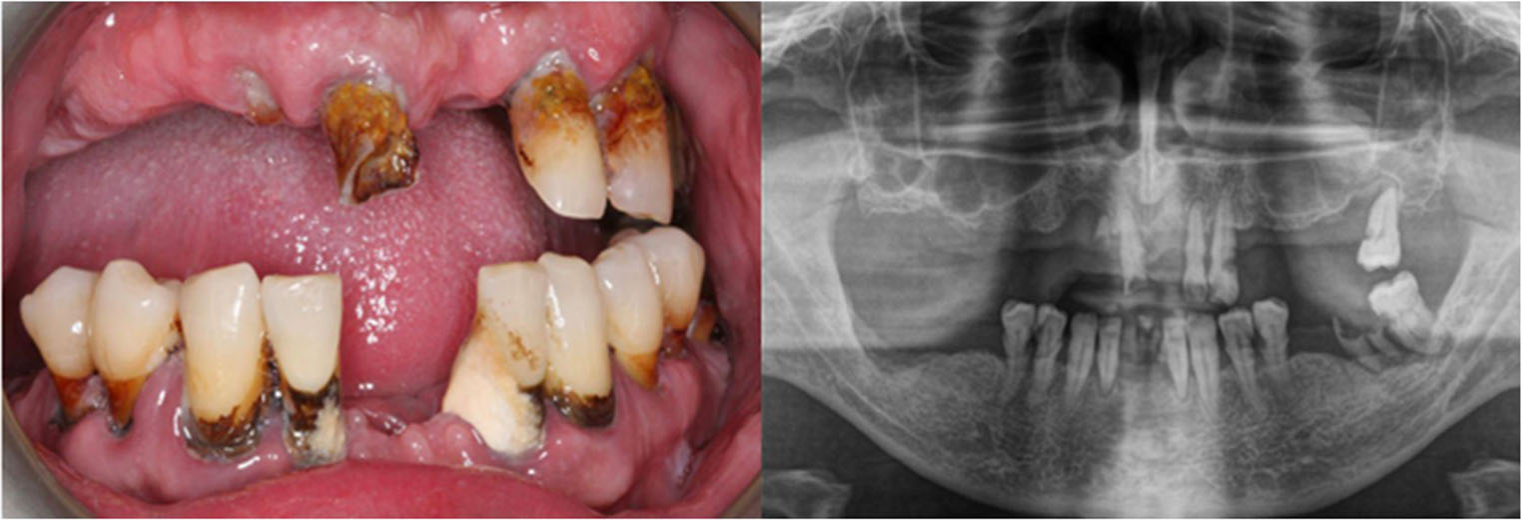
A 62-year-old man with advanced periodontal disease. Visible bone loss in the radiographic image. In the clinical picture, tartar deposits above and below the gingiva are visible
